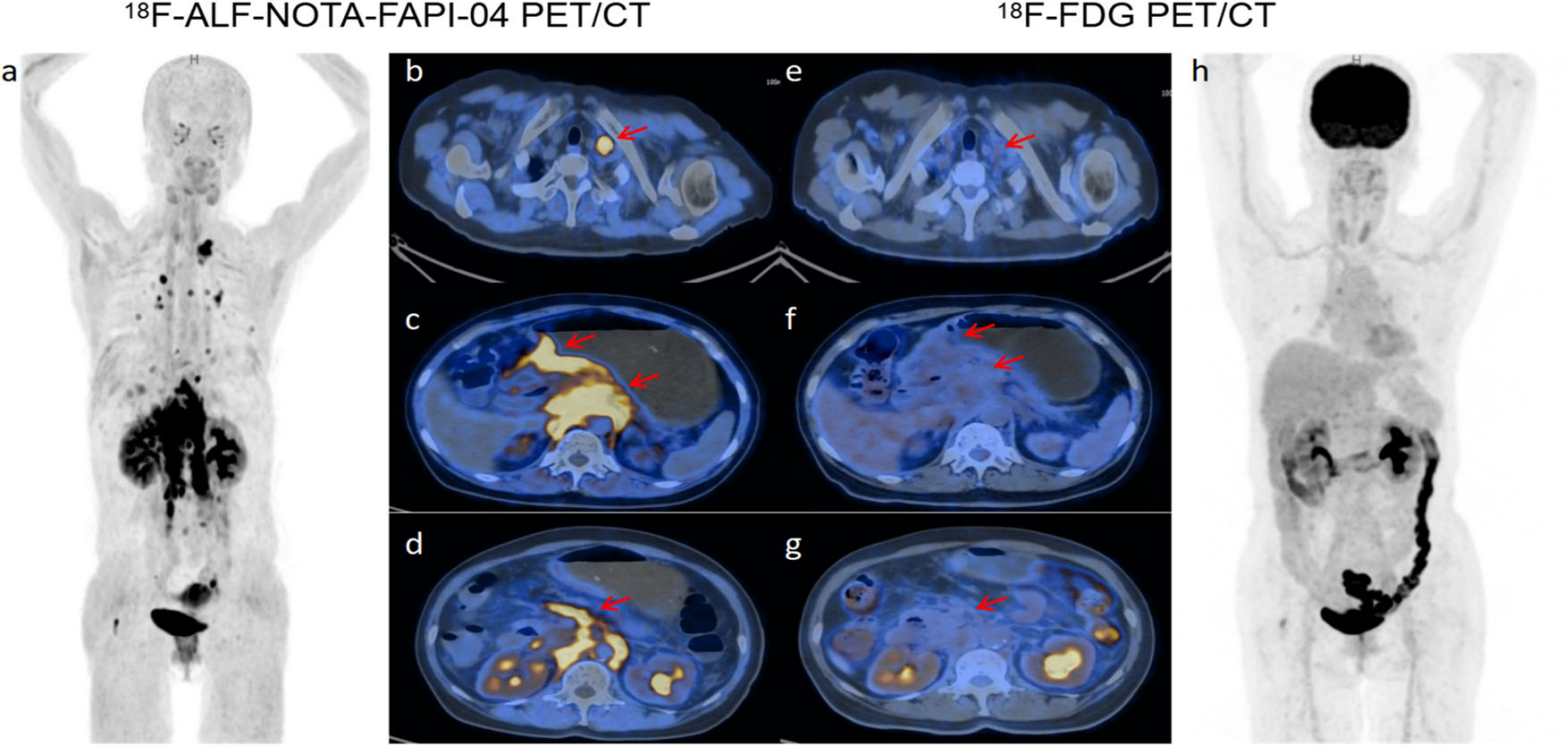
A 64-year-old female patient with signet ring cell carcinoma. The uptake of lesions in [^18^F]ALF-NOTA-FAPI-04 PET/CT (a–d) was higher than that in [^18^F]FDG PET/CT (e–h) (primary tumor SUVmax = 12.8 vs. 2.5; lymph node metastasis SUVmax = 25.0 vs. 1.8; peritoneal metastasis SUVmax = 26.5 vs. 3.1, respectively
